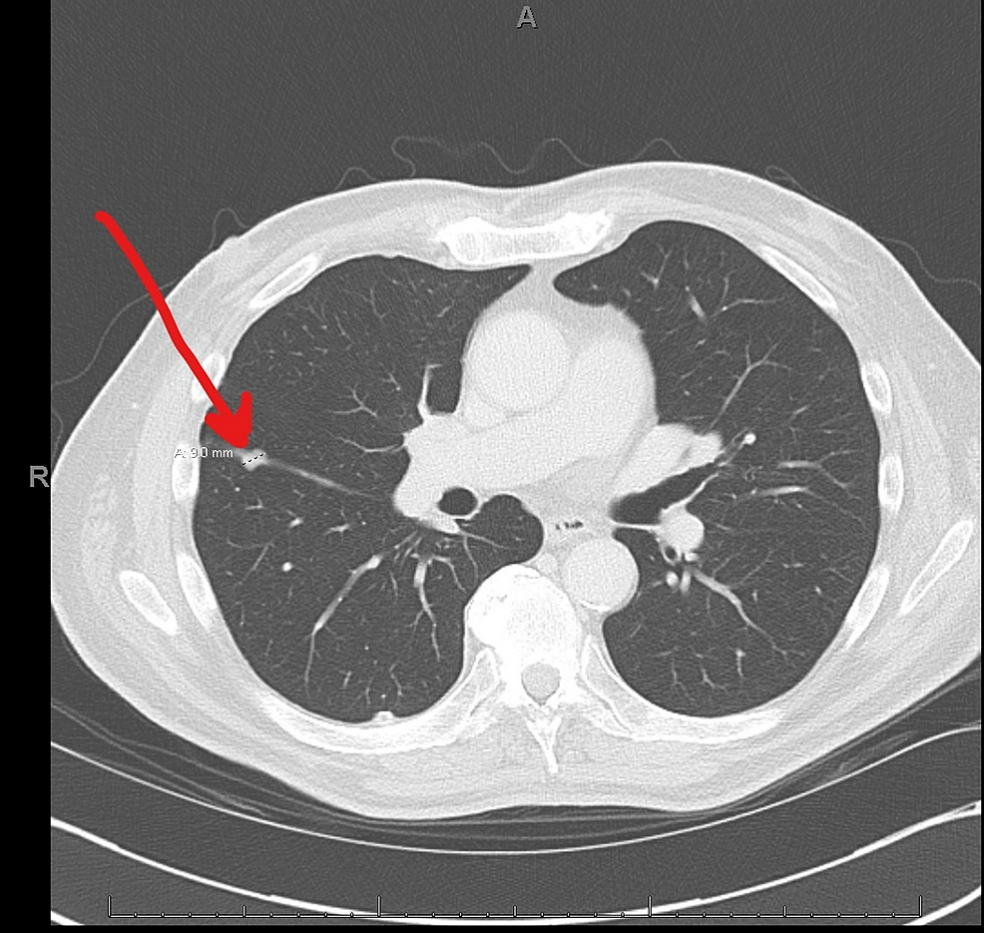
Preoperative chest CT following neoadjuvant chemotherapy demonstrating 9 mm nodule in the right lung CT: computed tomography.
